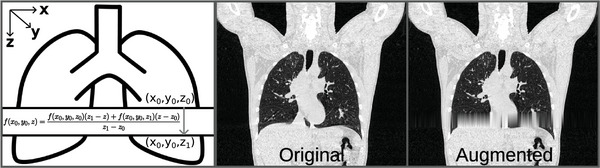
Artificial interpolation artifacts are created using linear interpolation. Stacks of data are removed from an image. Points from the top and bottom of the stack are linearly interpolated to generate the artifact. (Left) Mathematical equation and visual aid for applying the artificial artifact. (Middle) Original CT image. (Right) The same CT image with an artificial artifact.
